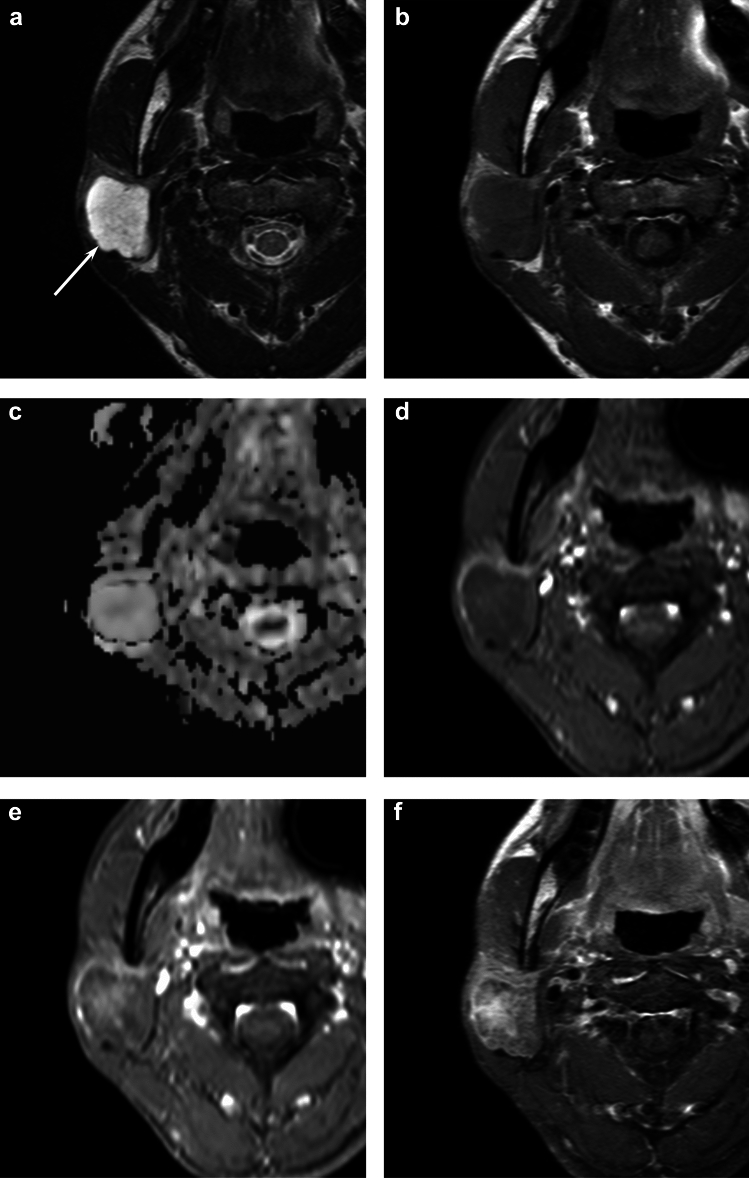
A 34-year-old woman with pleomorphic adenoma of the right parotid gland. a T2-weighted image shows a heterogeneously marked hyperintense lesion with lobulated margin (arrow). b T1-weighted image shows heterogeneously hypointense relative to the spinal cord. c ADC map shows extremely high ADC value (2.41 × 10^−3^ mm^2^/s). d Fat-suppressed dynamic contrast-enhanced T1-weighted image 30 s after intravenous administration shows minimal enhancement. e Fat-suppressed dynamic contrast-enhanced T1-weighted image 180 s after intravenous administration shows gradual enhancement. f Fat-suppressed contrast-enhanced T1-weighted image shows mild enhancement
